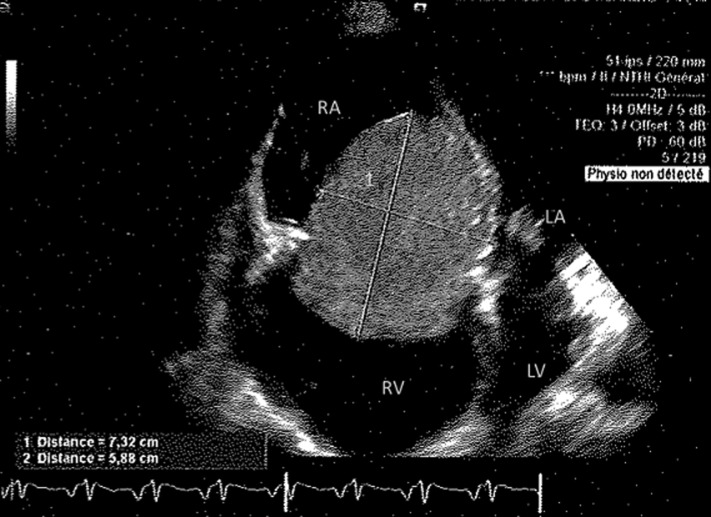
transthoracic echocardiography showing a right atrial mass passing through the tricuspid valve; 1: voluminous right atrial mass, RA: right atrium, RV: right ventricle, LA: left atrium, LV: left ventricle
